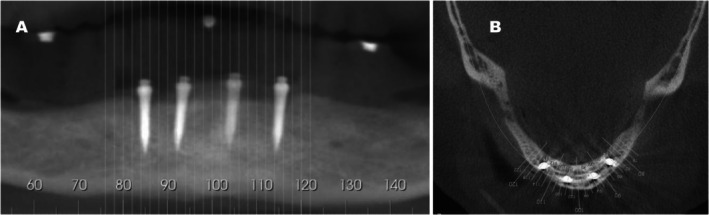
(A) Panoramic CBCT image with the mini implants in position and the three denture teeth landmarks. (B) Sagittal CBCT view.
